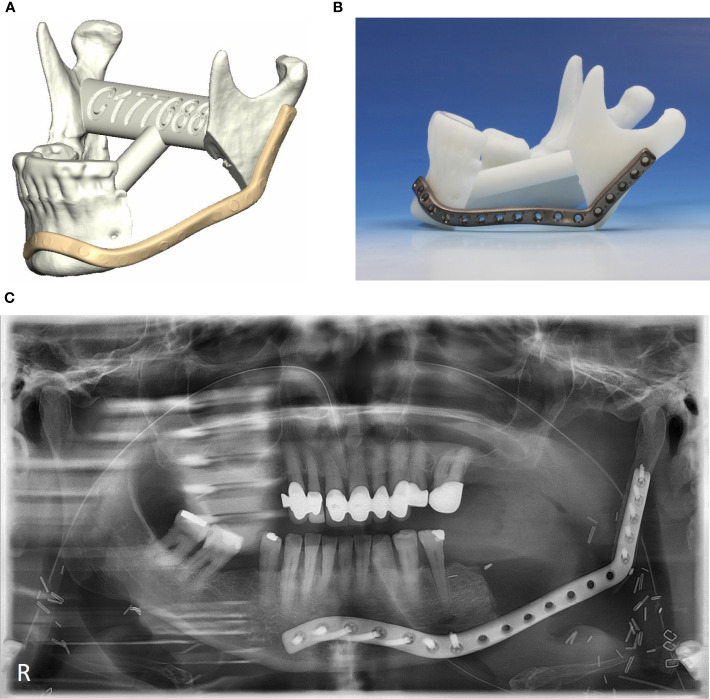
Non-complex patient-specific implants. (A) Digital planning, (B) patient-specific implant on plastic model, (C) postoperative orthopantomogram.
